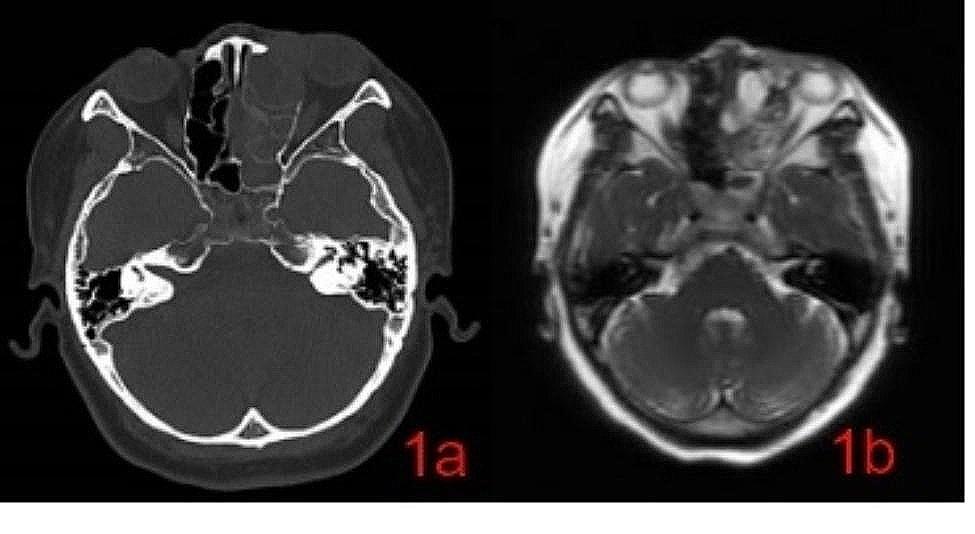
a: CT: The presence of erosion in the left sinus wall, the cricoid plate, and the left intraorbital wall. b: MRI: The mean signal of the mass on the t2-weighted sequence was high signal, with unclear boundaries and large invasion range
